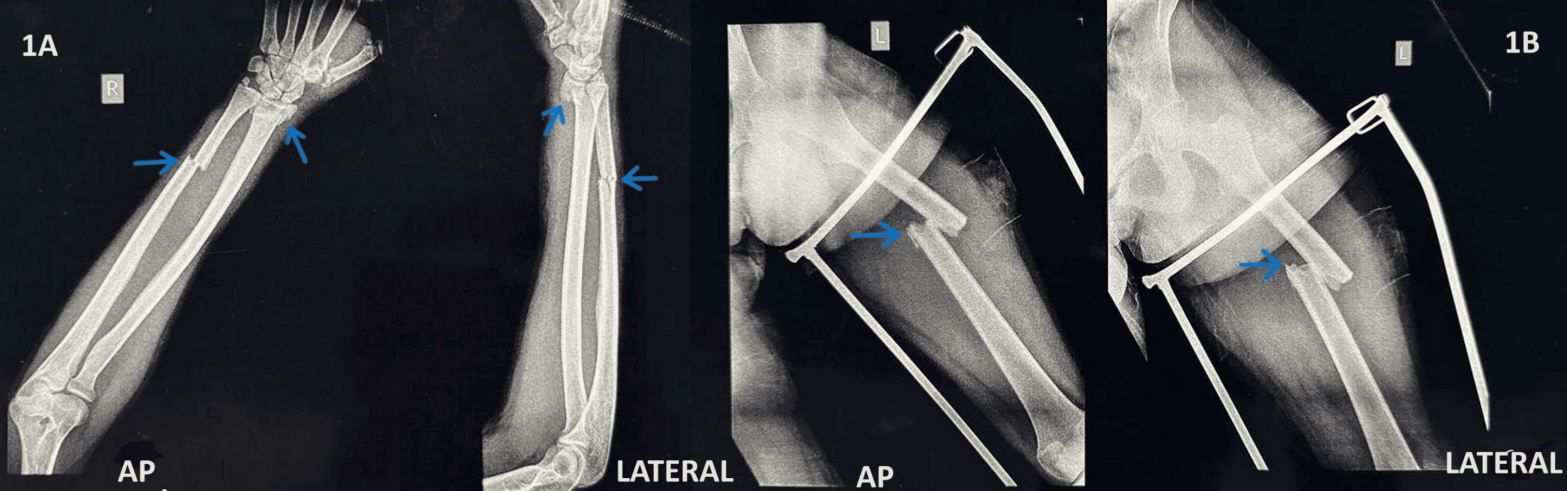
Preoperative plain radiograph. (A) Arrows showing right distal end radius fracture and distal one-third ulna shaft fracture in anteroposterior (AP) and lateral views. (B) Arrows showing left femur shaft fracture in AP and lateral views.
